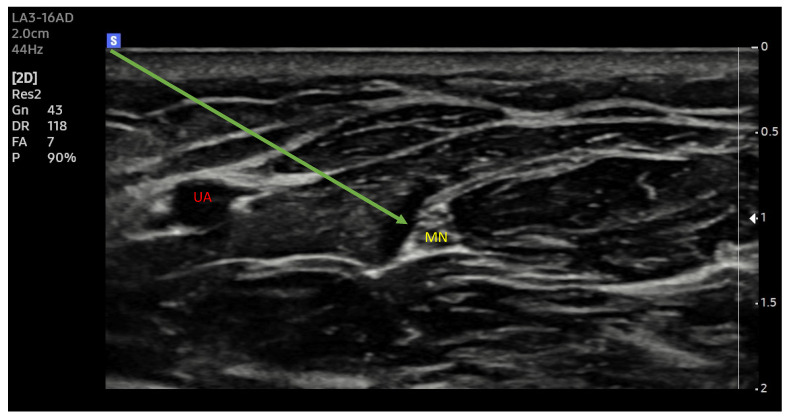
Median nerve. Ultrasound transverse view. MN—median nerve, UA—ulnar artery; Arrow —needle trajectory; Arrowhead—extraneuronal needle placement.
